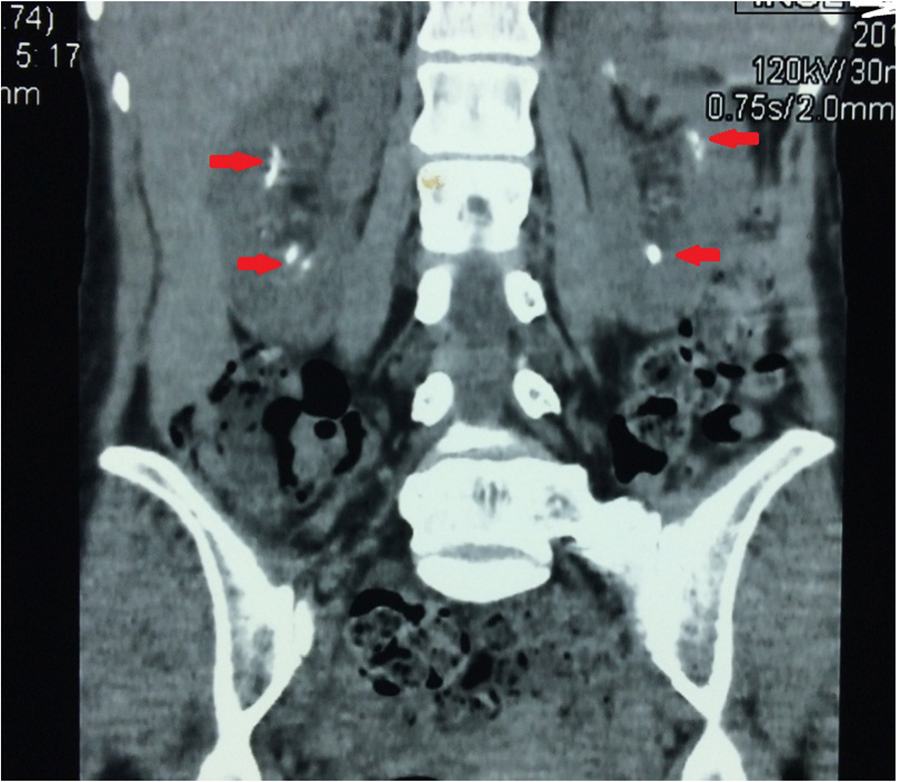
Non-contrast CT kidneys showing bilateral nephrolithiasis
